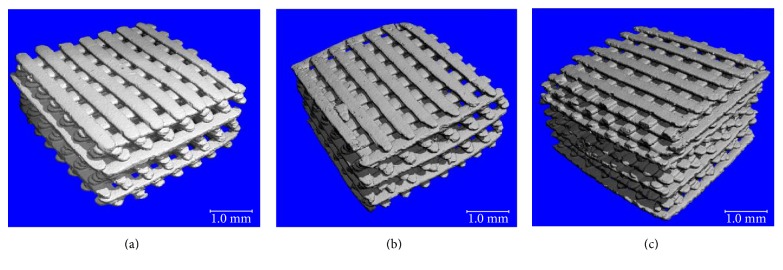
3D images of the scaffolds by microcomputed tomography (*μ*CT): 12.5 *μ*m scaffold (a), 25 *μ*m scaffold (b), and (c) 50 *μ*m scaffold (c).
